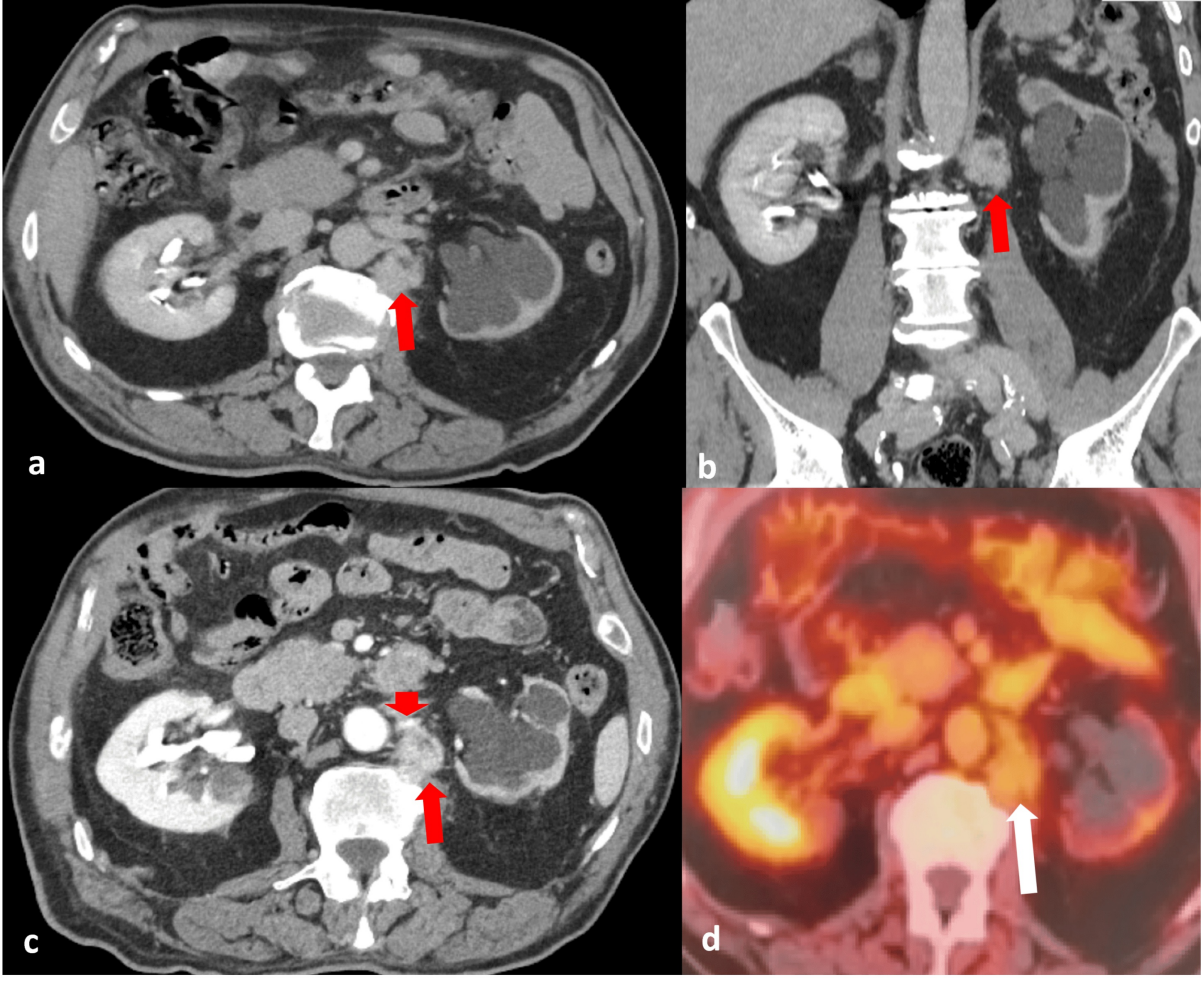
(a) Relatively well-defined lesion at the proximal left renal artery (arrow) in axial view (portal-venous phase) and (b) in coronal view (portal-venous phase). (c) Arterial phase showing the same relatively well-defined, heterogeneously enhancing lesion at the proximal left renal artery (arrow) in axial view. Note the lesion’s close proximity to the left renal artery (arrowhead). (d) PET-CT image of the same lesion at the left renal artery (arrow), measuring 2.2 × 1.8 cm with an SUVmax of 2.8. SUVmax: Maximum Standardized Uptake Value.
